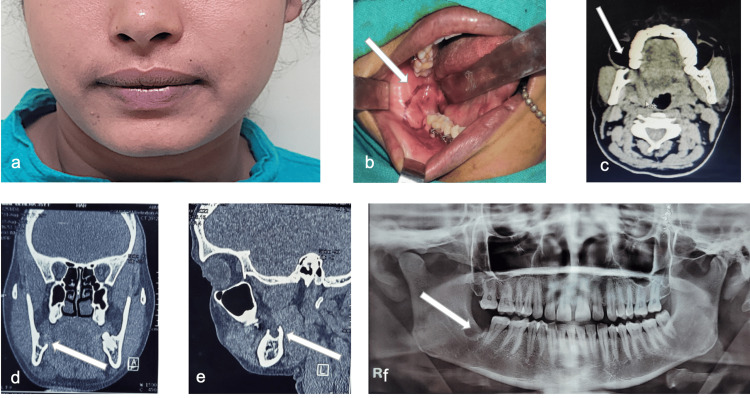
a: Extra oral image. b: Intraoral image. c-e: Axial, coronal, and sagittal CECT sections showing an osteolytic lesion. f: Preoperative OPG CECT: Contrast-enhanced computed tomography; OPG: orthopantomogram
